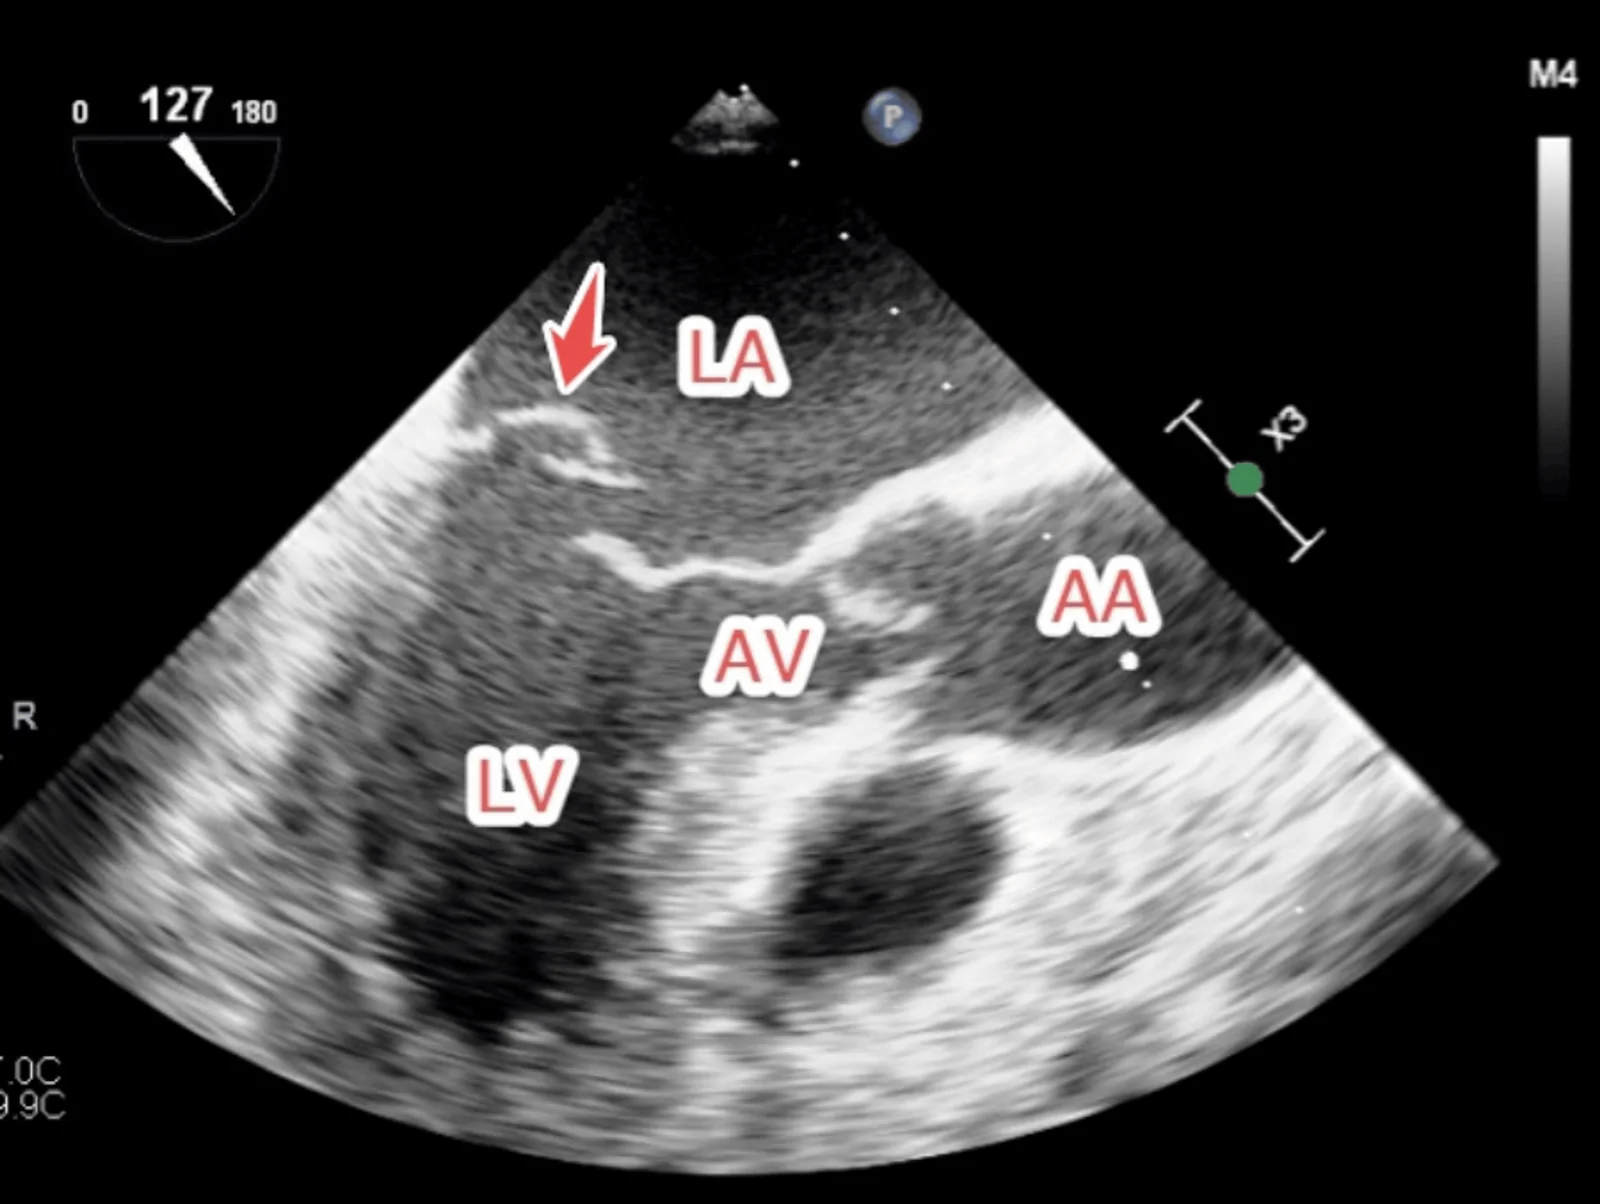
Transesophageal echocardiographic demonstration of P2 posterior mitral leaflet flail due to primary chordae tendineae rupture. The arrow highlights the flail P2 segment resulting from ruptured primary chordae tendineae. AA: ascending aorta; AV: aortic valve; LA: left atrium; LV: left ventricle.
